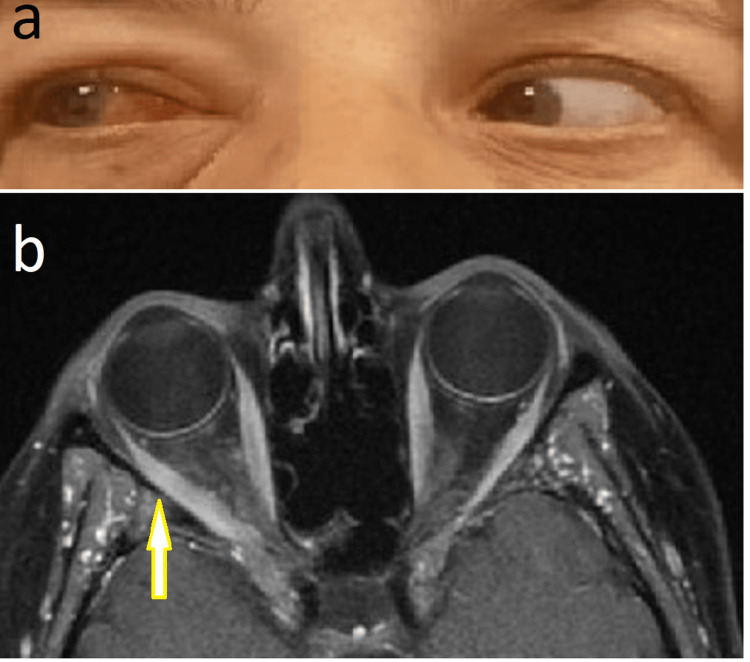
a) Mild limitation of abduction in the right eye, with conjunctival hyperemia; b) Moderate enlargement of the right lateral rectus muscle on MR imaging.
